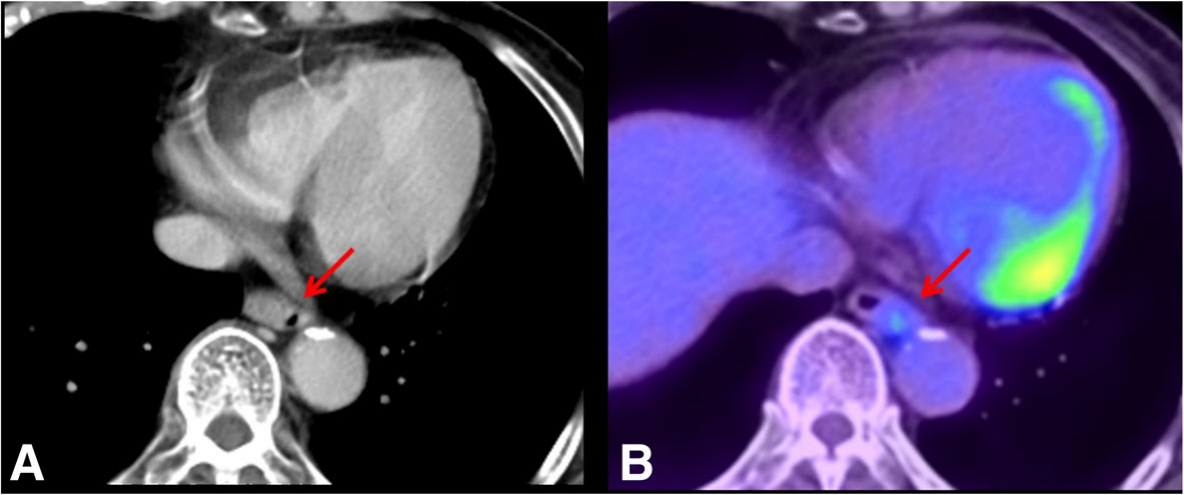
Findings of computed tomography (CT) and 18F–fluorodeoxyglucose (18F–FDG) positron-emission tomography/computed tomography (PET-CT). a A transverse plane of an enhanced CT scan shows an intraluminal mass in the lower esophagus, which is well-defined without infiltration of the surrounding tissue (arrows). b 18F–FDG PET-CT scan shows 18F–FDG uptake at the same lesion of the lower esophagus; the SUV max of the lesion is 3.1 (arrows)
